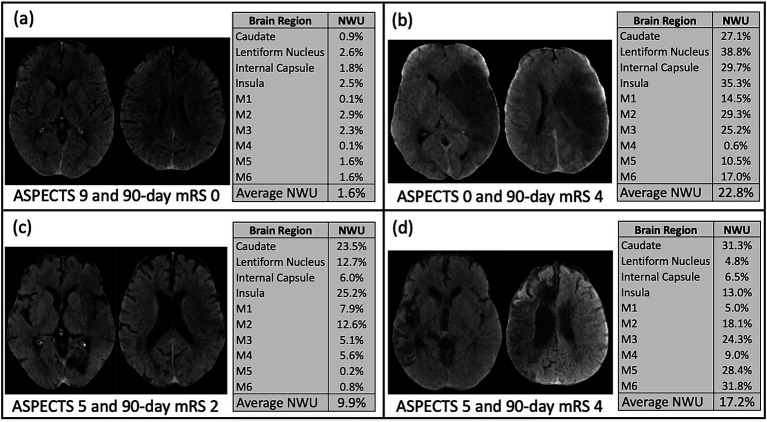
These four examples from the study cohort demonstrate common clinical scenarios. (a) Subject with ASPECTS 9, average NWU 1.6%, and 90-day mRS 0; (b) Subject with ASPECTS 0, average NWU 22.8%, and 90-day mRS 4; (c) Subject with ASPECTS 5, average NWU 9.9%, and 90-day mRS 2; (d) Subject with ASPECTS 5, average NWU 17.2%, and 90-day mRS 4.
